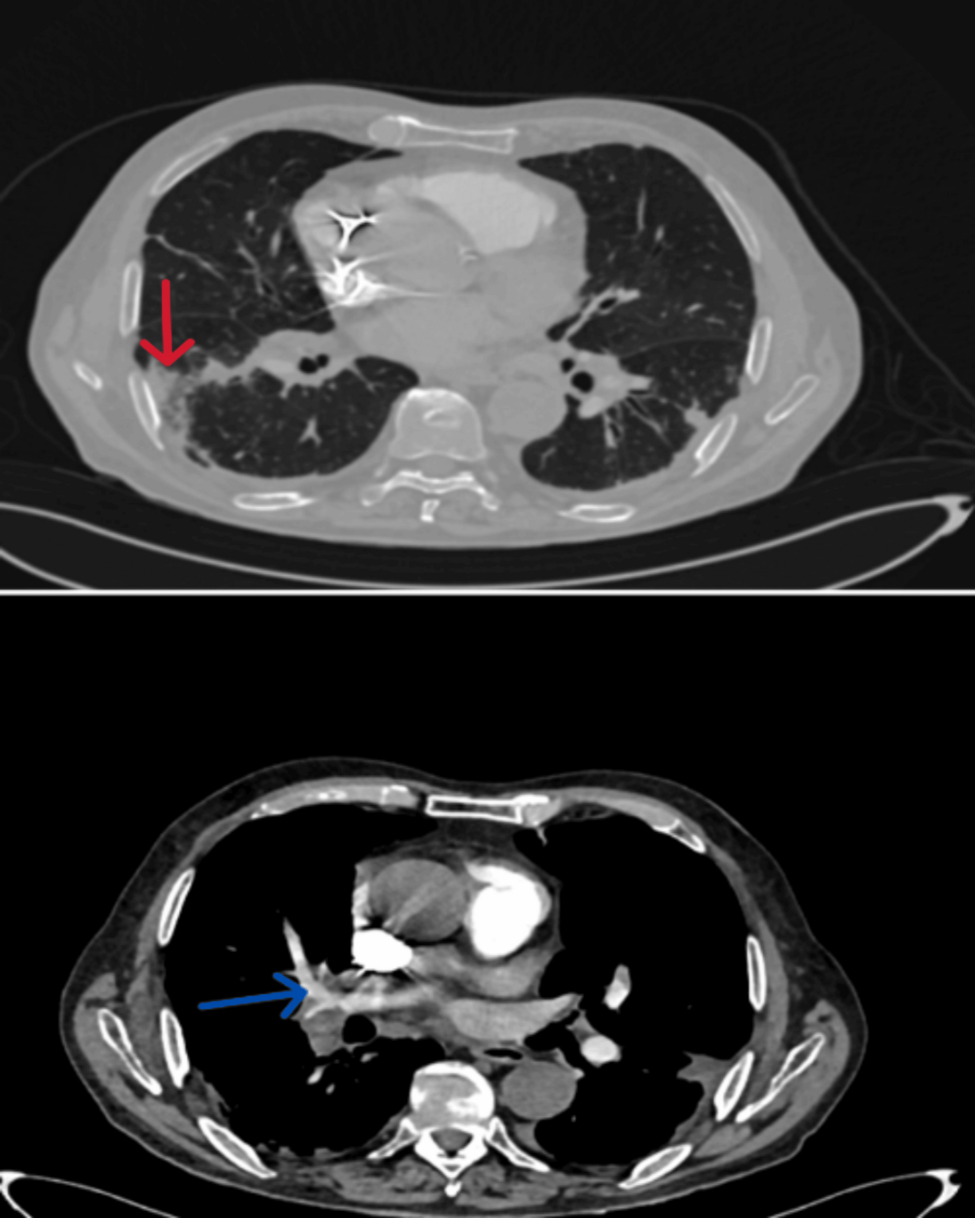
CT scan of bilateral pulmonary emboli with thrombotic masses in the right pulmonary artery and lower lobe branches. Consolidated focal infiltrates in the right lower and left upper lobes. Red arrows: Consolidated focal infiltrates, Blue arrows: Bilateral pulmonary emboli with thrombotic masses in the right pulmonary artery and lower lobe branches
